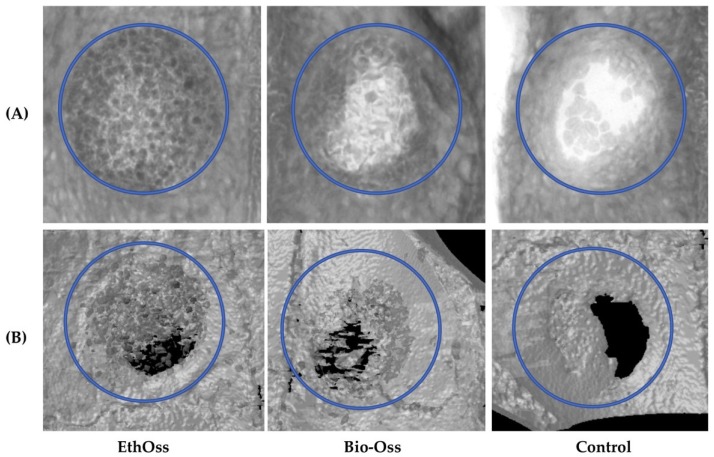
(A) Axial sections and (B) reconstructed three-dimensional (3D) micro-computed tomography (CT) images of the 8-mm-diameter defect sites after eight weeks of healing.
